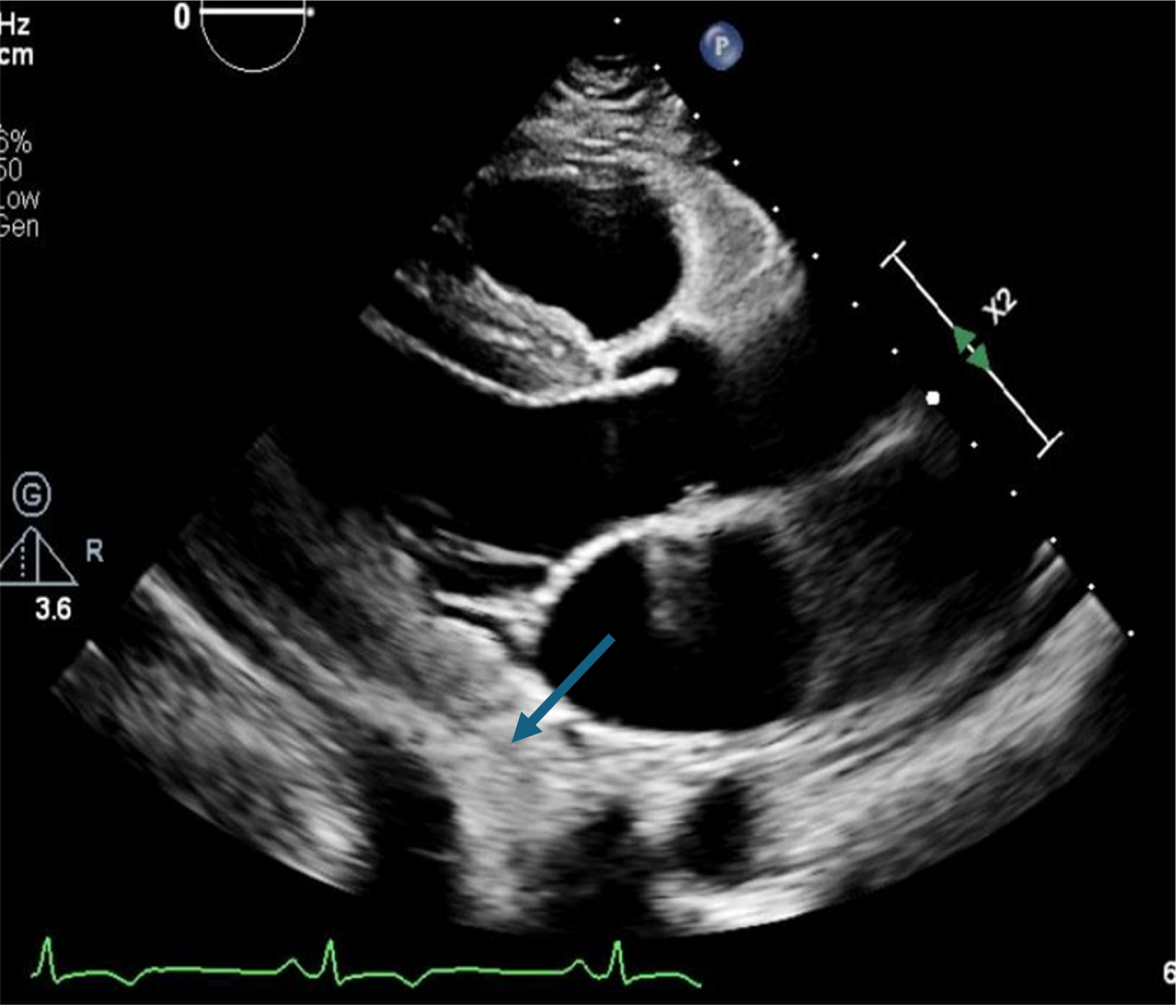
TTE. Parasternal long axis view showing decrease in size of pericardial effusion to trace(arrow) after treatment in follow up.
